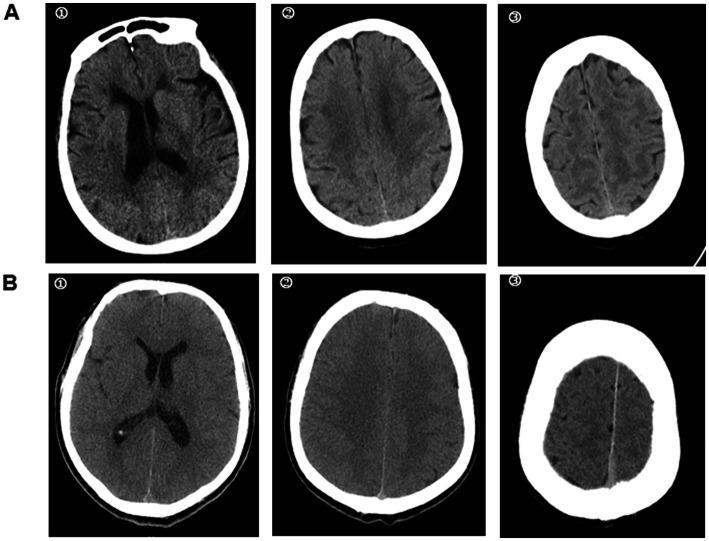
Cranial CT in heat stroke patients with different neurological outcome. A heat stroke patient was admitted to the hospital. Cranial CT in the emergency department showed a well-defined grey-white matter difference in the brain. He was discharged from the hospital with CPC 1 (A). While, the cranial CT in another heat stroke patient suggested cerebral oedema with loss of grey-white matter discrepancy. The CPC was 5 at discharge from hospital (B). ① basal ganglia level; ② centrum semiovale level; ③ high convexity level.
